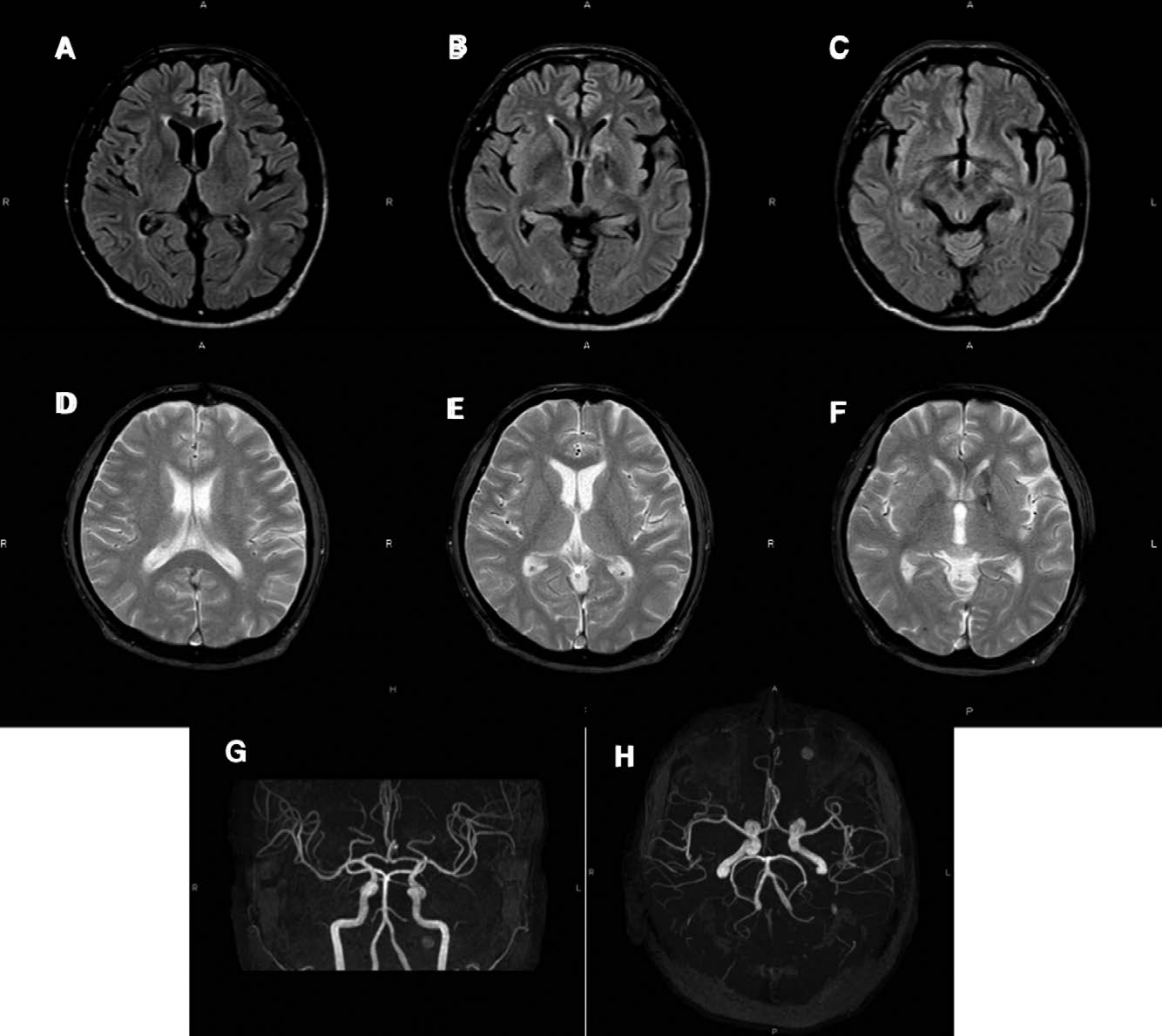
Axial FLAIR images acquired on hospital day 58 show no brain abscess or hemorrhagic enlargement (A–C). The amount of hemorrhage along the intracerebral route of the gimlet on axial T2* imaging is less than on hospital day 5 (D–F). There is no evidence of traumatic cerebral aneurysm formation (G and H).
